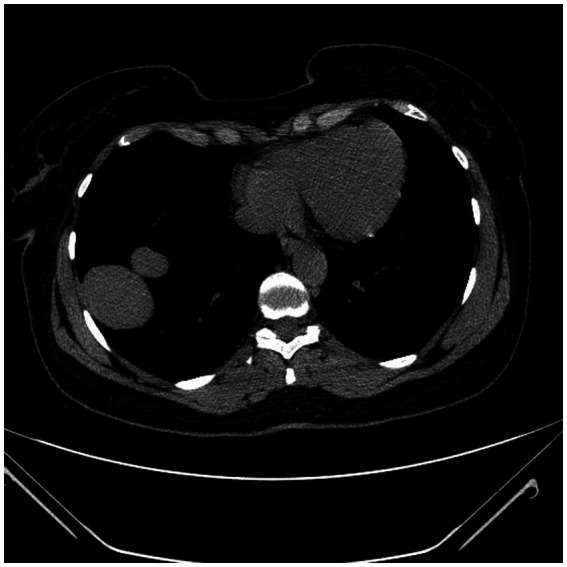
Axial CT image showing right diaphragmatic defect with herniation of the liver into the thoracic cavity.
